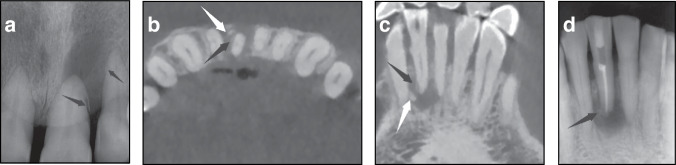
Characteristic radiographic images of teeth with cemental tear. a Periapical radiographs of 21 and 22 presented the “prickle-like” radiopaque masses detached from the root surface (black arrows). b–d CBCT and periapical radiographs of tooth 41 with cemental tear. Reconstructed CBCT images of 41 in b coronal and c sagittal views, the black arrow indicating the “flake-like” radiopaque mass detached from the disto-lateral aspect of the root with periapical radiolucent lesions, and the white arrow indicating normal alveolar bone. However, periapical radiograph of 41 d after RCT presented with an irregular radiopaque mass (black arrow), which was blurry and easily overlooked, near the disto-lateral aspect at the apical third of the root and periapical radiolucent lesions
